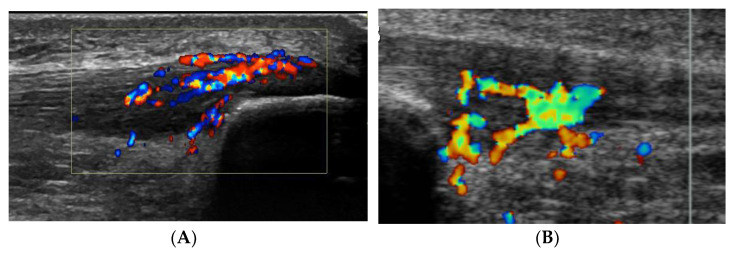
(A,B). Ultrasound and Doppler picture from 2 patients suffering from chronic painful patellar tendinopathy. There is a thickened proximal patellar tendon, including irregular tendon structure, hypo-echoic regions and high blood flow on the dorsal side of the tendon. (A): This patient also had severe tenderness on the superficial side of the proximal tendon, where there was a localized thickening of the paratenon including high blood flow. (B): This patient had no tenderness on the superficial side of the proximal tendon, where there was a normal paratenon and normal blood flow.
